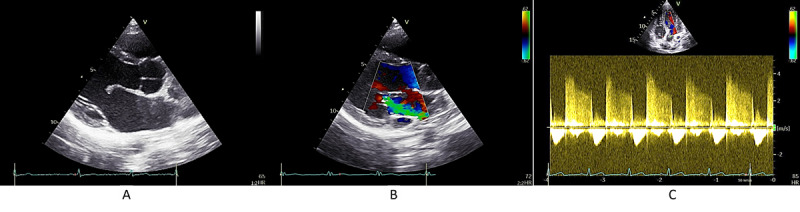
Pathological findings based on World Heart Federation 2012 criteria [3] for a patient with rheumatic heart disease: (A) parasternal long axis (PLAX) view showing doming of anterior mitral leaflet and restricted posterior mitral leaflet, (B) mitral regurgitation via color Doppler imaging in PLAX view, and (C) aortic regurgitation in continuous-wave Doppler imaging. The mitral valve is shown using an arrow.
